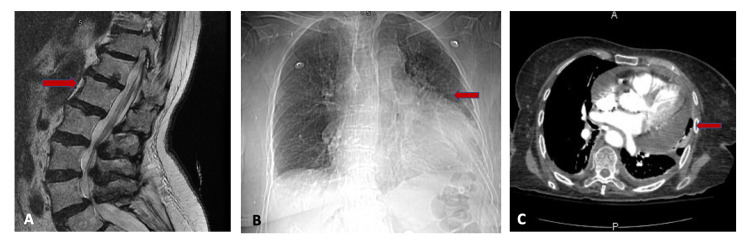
(A) MRI lumbar spine is showing degenerative and stenotic changes. CT pulmonary angiogram is showing cardiomegaly (B) and large pericardial effusion (C).
